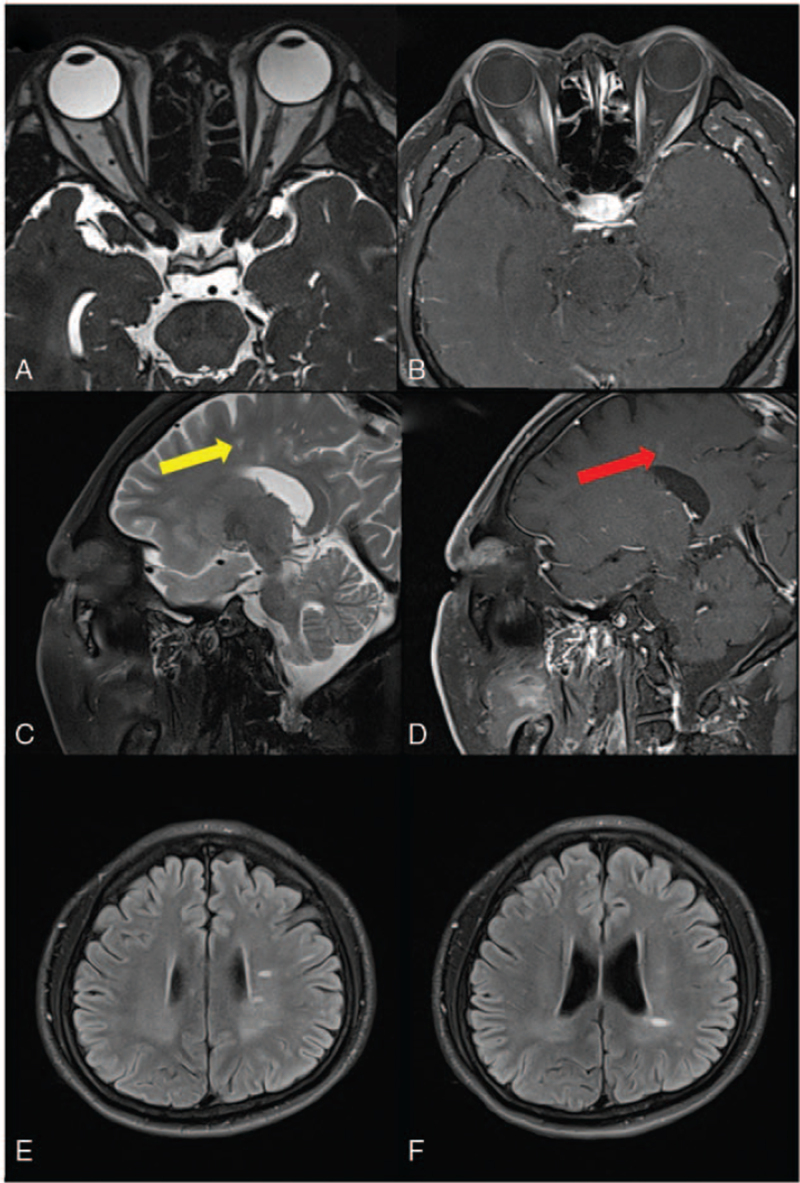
Optic nerve and brain magnetic resonance image findings during the first attack. (A) Axial constructive interference in steady state T2-weighted imaging showed high-signal intensity lesion of right optic nerve. (B) Axial postgadolinium T1-weighted MRI demonstrated enhancement of the right optic nerve in the intraorbital segment. (C, D) Sagittal T2-weighted and postgadolinium T1-weighted images showed several gadolinium-enhancing (red arrow) and nonenhancing (yellow arrow) lesions. (E, F) Axial fluid-attenuated inversion recovery images showed several high-signal intensity lesions in the left periventricular region. MRI = magnetic resonance imaging.
